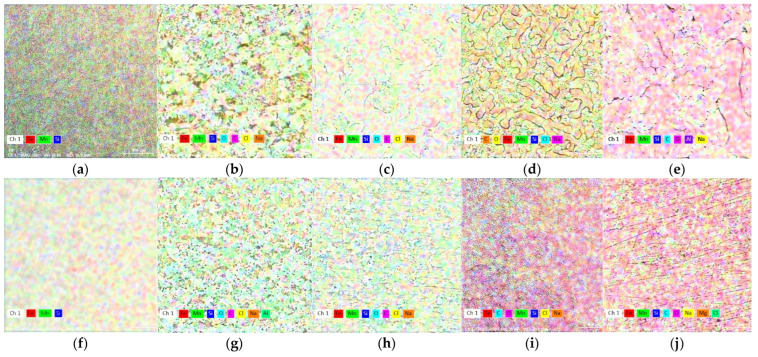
X-ray mapping of chemical elements on the surface. The initial samples, cast (a) and wrought (f), (b); C sample after 1 day immersion and (c) after ultrasound cleaning; (d) C sample after 7 days immersion and (e) after ultrasound cleaning; (g) W sample after 1 day immersion and (h) after ultrasound cleaning; (i) W sample after 7 days immersion and (j) after ultrasound cleaning.
